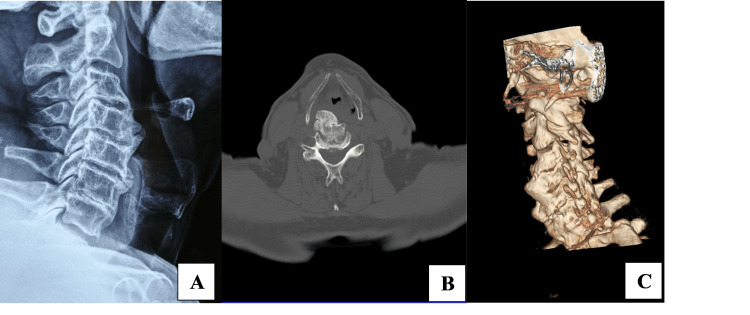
The presence of cervical spine osteophytes in a) cervical spine X-ray, b) 3D reconstruction computed tomography, and c) computed tomography
